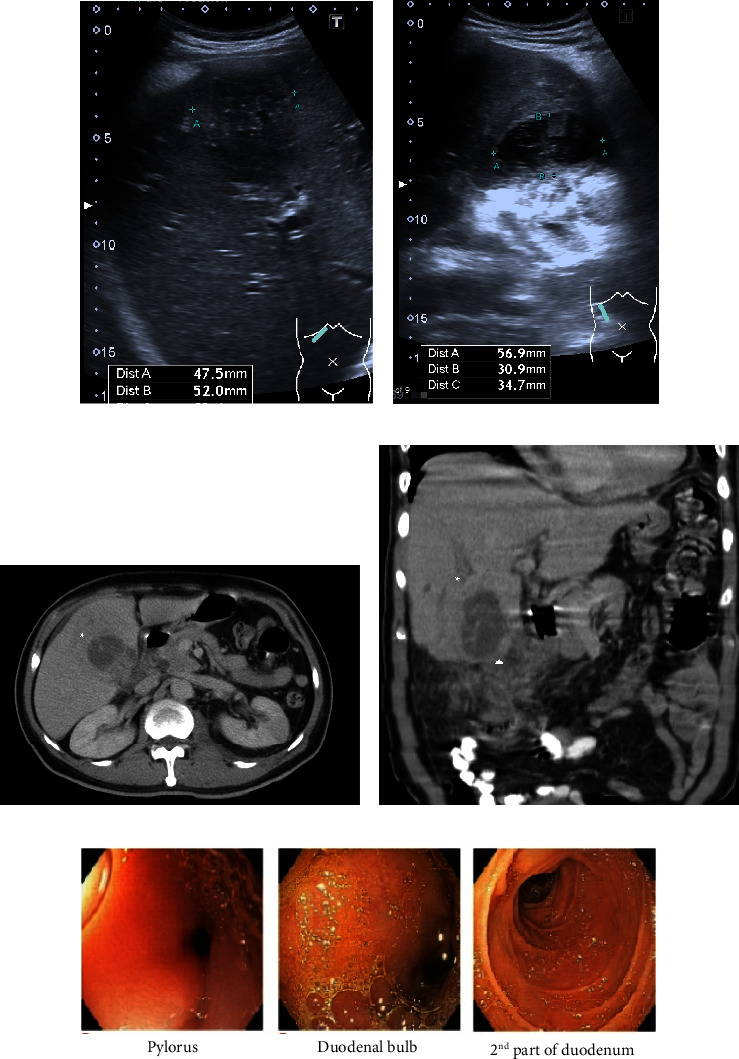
Preoperative investigations. (a, b) Ultrasonography of abdomen showed a heteroechoic gallbladder mass with suspected liver invasion. (c, d) CT whole abdomen revealed a hypodensity mass replacing the gallbladder, invading segment 4, 5 of the liver (asterisk), right-sided colon (triangle) and suspected duodenal invasion. (e) EGD findings showed no mucosal-invading lesions in stomach and duodenum.
